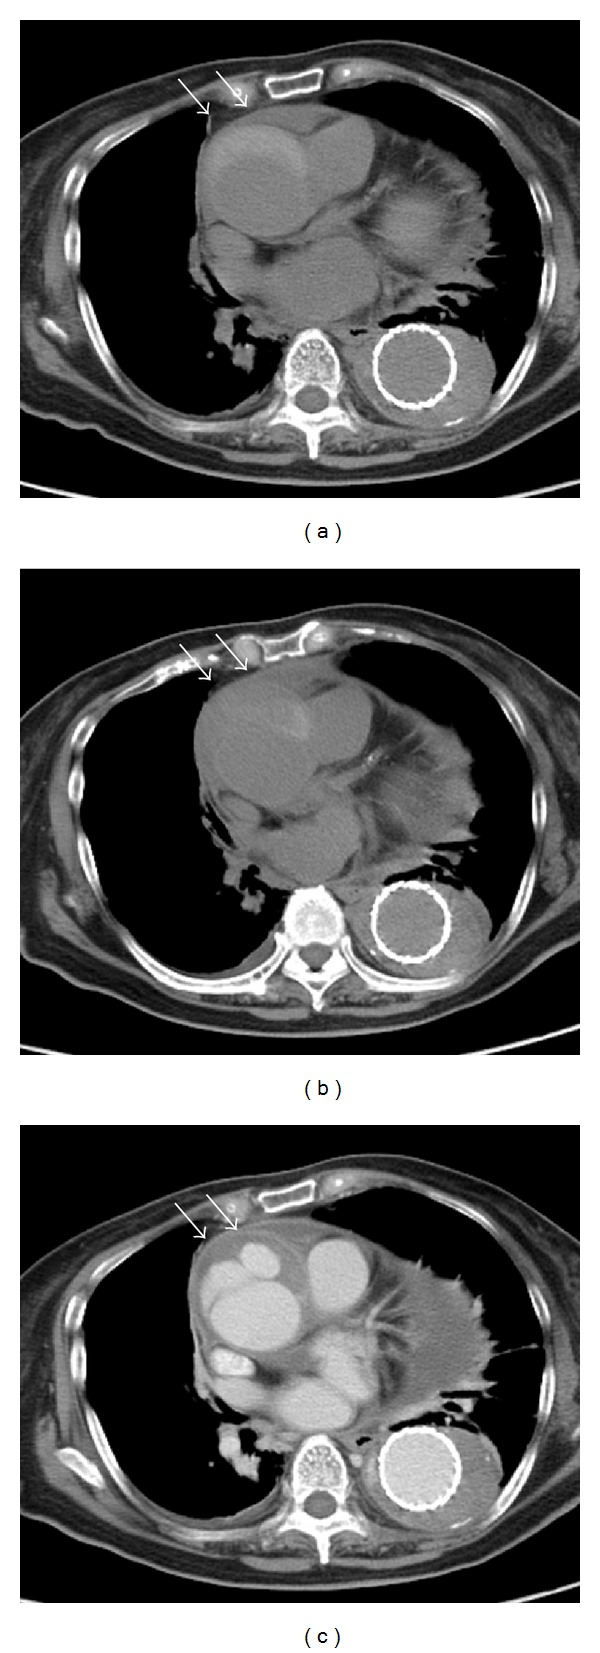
Computed tomography of the chest. (a) Plain computed tomography (CT) performed on the first day. The density of the false lumen (white arrows) of the ascending aorta was homogenous and higher than that of the true lumen and that of the false lumen of the descending aorta, indicating relatively newly developed thrombosis. The maximum diameter of the ascending aorta was 55 mm. (b) Plain CT performed on the 27th day. The density of the false lumen of the ascending aorta was partially heterogeneous compared to that observed on the first day. (c) Contrast-enhanced CT performed on the 29th day. The false lumen of the ascending aorta was also enhanced by contrast medium, directly indicating ongoing aortic dissection. The amount of pericardial effusion was also increased. The maximum diameter of the ascending aorta was enlarged (60 mm), indicating an increased risk for rupture of the ascending aorta.
